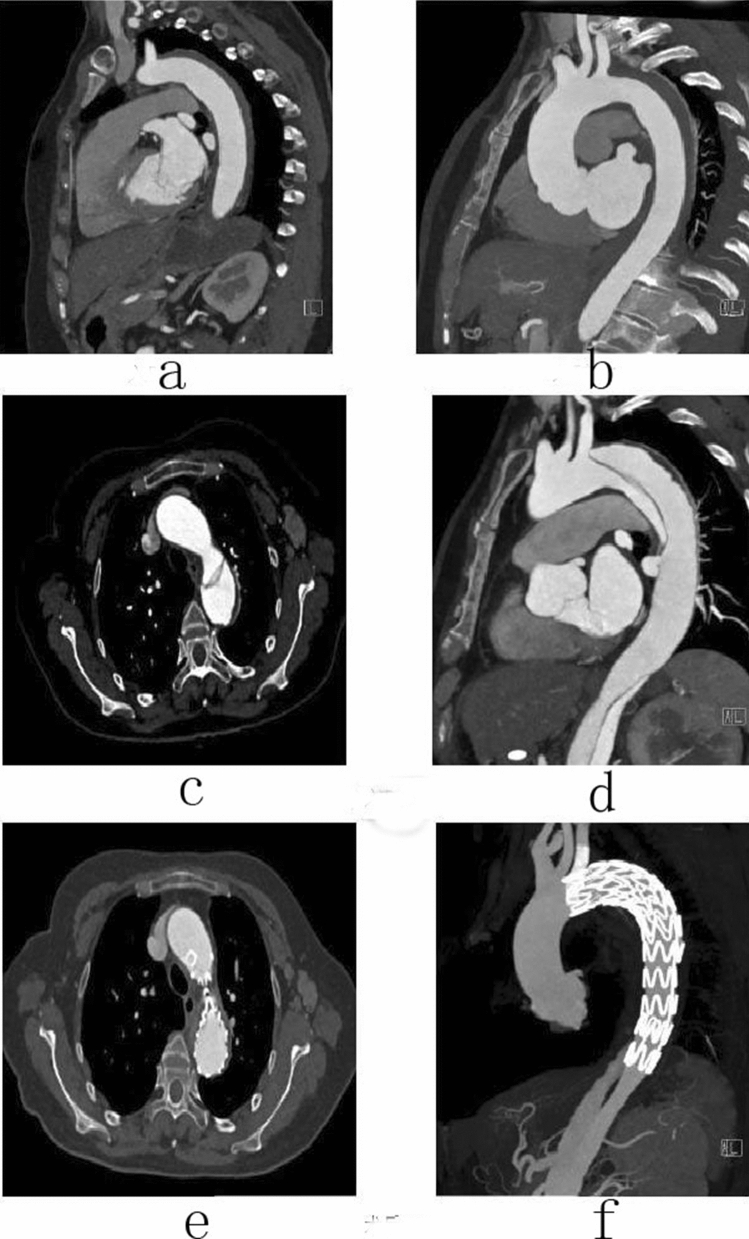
Female, 58 years old, admitted to hospital for “sudden pain in chest for 6 h”, Diagnosis: Stanford type B aortic dissection; Treatment: IMH TEVAR treatment. CTA of the patient in different periods: (a) Admission; (b) 5 days after admission; (c) 16 days after admission; (d) 16 days after admission; (e) 7 days after surgery; (f) 7 days after surgery.
